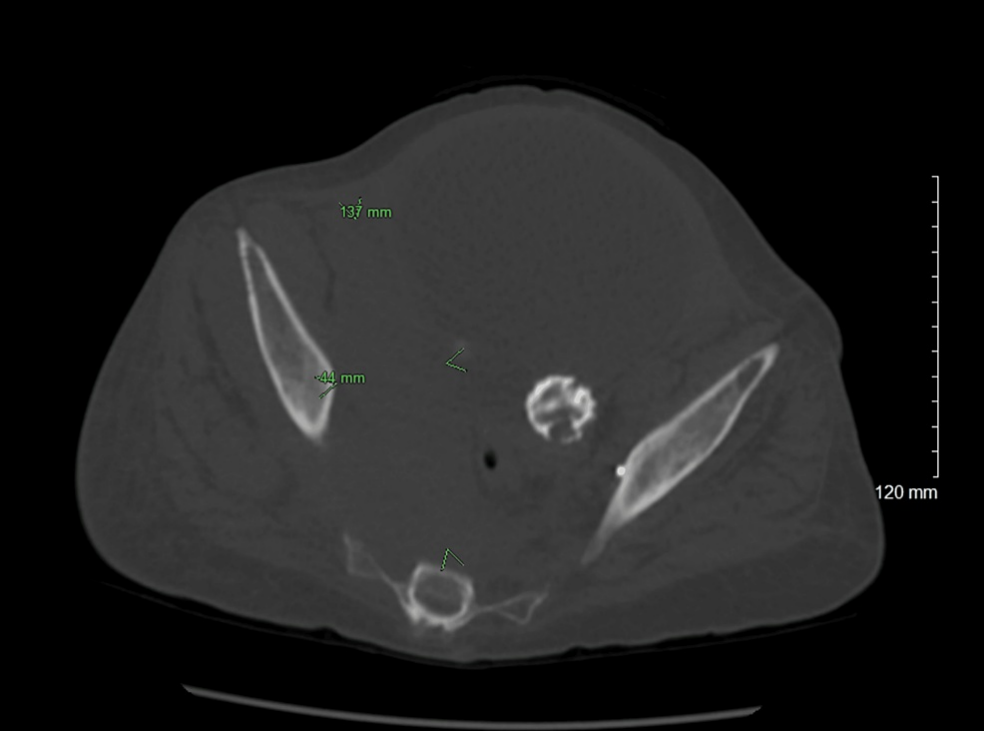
Non-contrast computed tomography of the pelvis demonstrating 4.4×13.7 cm mass (green arrowhead)
